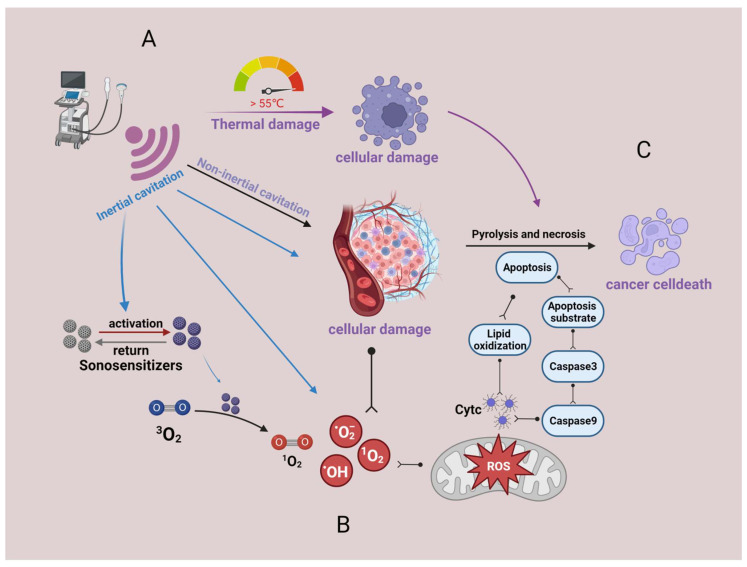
Principles of SDT for oncology treatment. (A) Ultrasound carries acoustic energy that can produce both thermal and non-thermal effects in the tumor region. The thermal effect can directly cause cellular damage. Non-thermal effects mainly occur as cavitation effects. (B) ROS generation. Ultrasound induces intracellular ROS generation inside the tumor by producing cavitation effects and activating sonosensitizers. (C) ROS cause tumor cell death by inducing cellular autophagy and necrosis, among other pathways. Created with BioRender.com (accessed on 23 August 2023).
